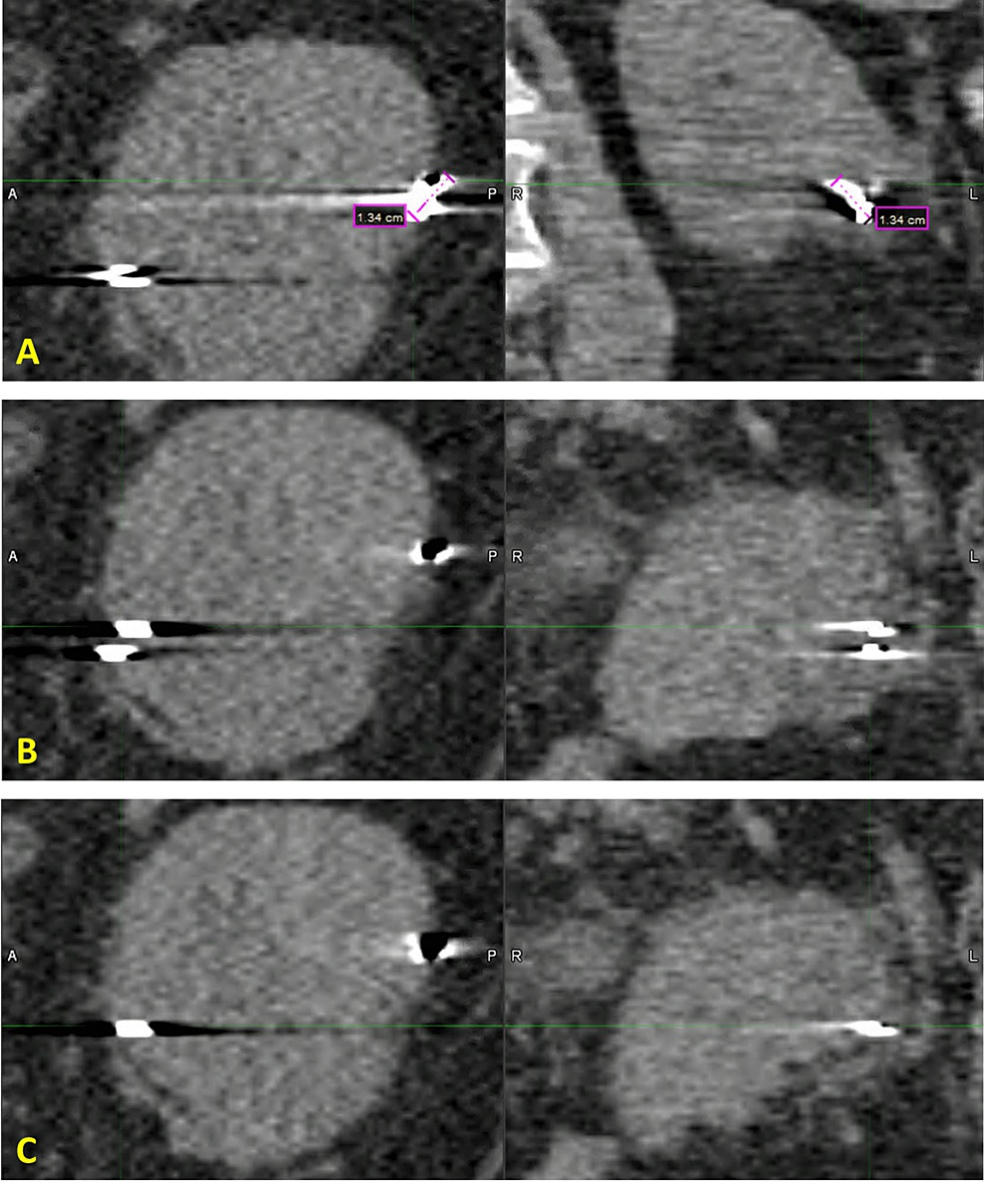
Renal case study 4DCT, sagittal and coronal views. (A) In the 80% phase, one fiducial appears to be 13mm long but this is more than double the known length of 5mm, suggesting it is from 4DCT artifact. (B) In the 90% phase, one fiducial appears to be two fiducials, but in the 80% phase (A) and 0% phase (C), it is confirmed to be a single fiducial; therefore it must be due to 4DCT artifact. This scan was not used in treatment planning. 4DCT: four-dimensional computed tomography
